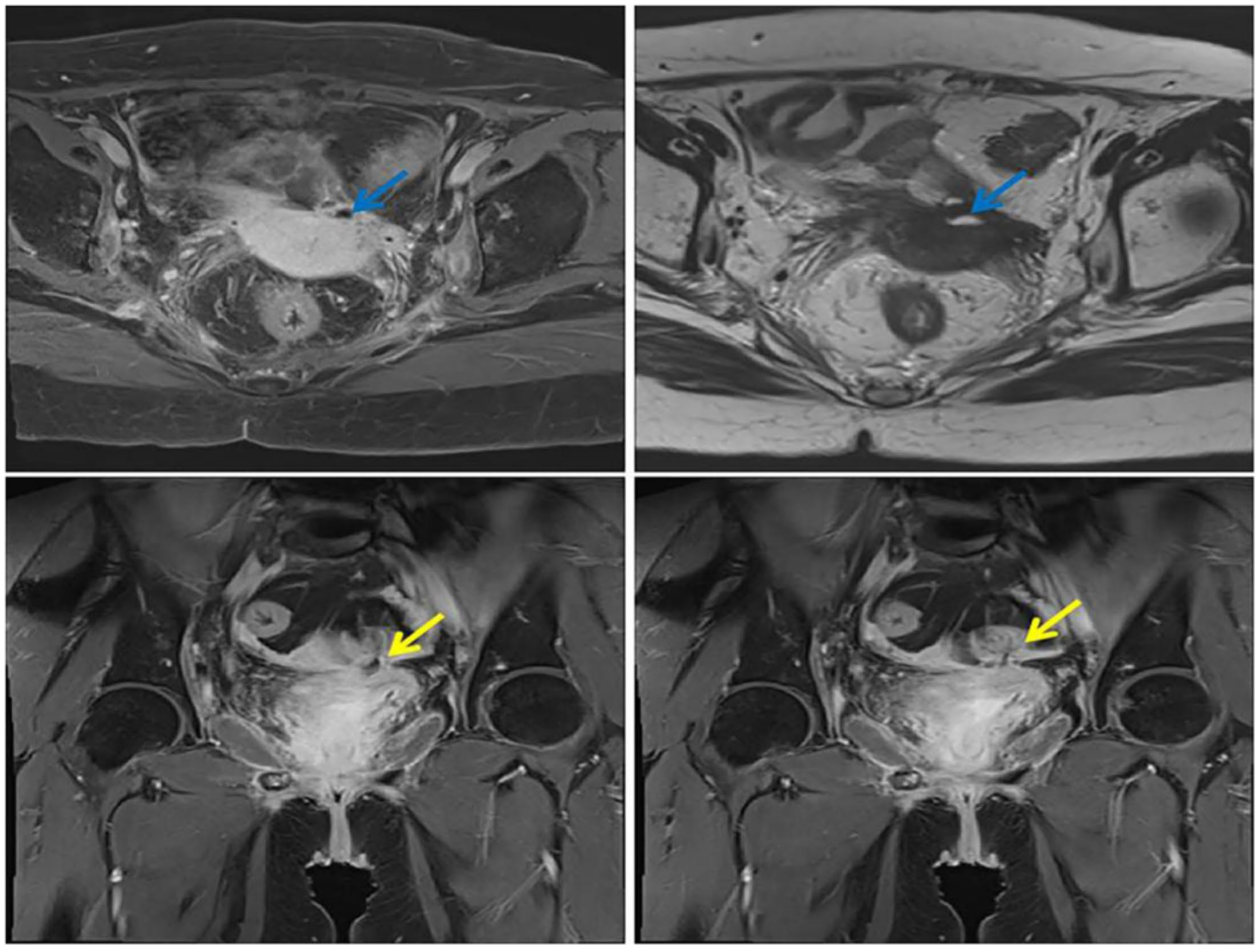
Pelvic MRI showing SVF (the blue arrow: axial Image; the yellow arrow: Coronal Image).
